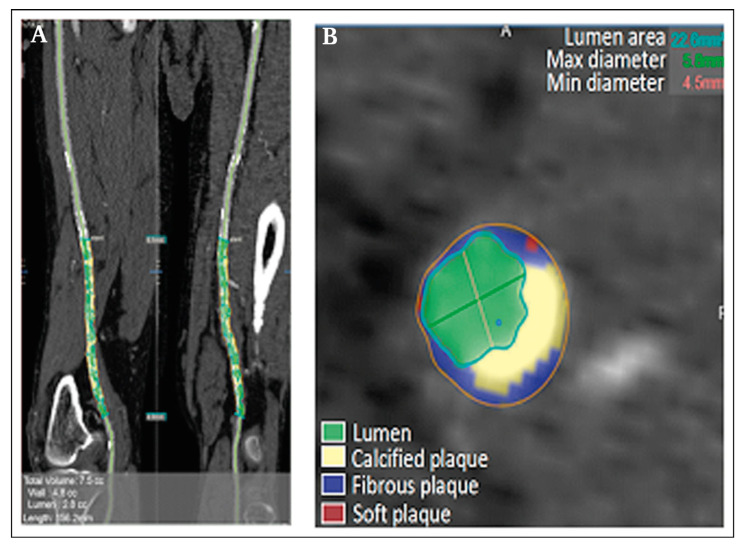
CT plaque tissue composition analysis of femoral artery plaque. (A) CTA images reconstructed in the volume-rendering mode; (B) automatic calculation of the calcified plaque volume within the cross-sectional image region of interest. The blue region indicates fibrous plaque; the green region indicates lumen; the red region indicates soft plaque; the yellow region indicates calcified plaque. Image reprinted from He et al. [31].
